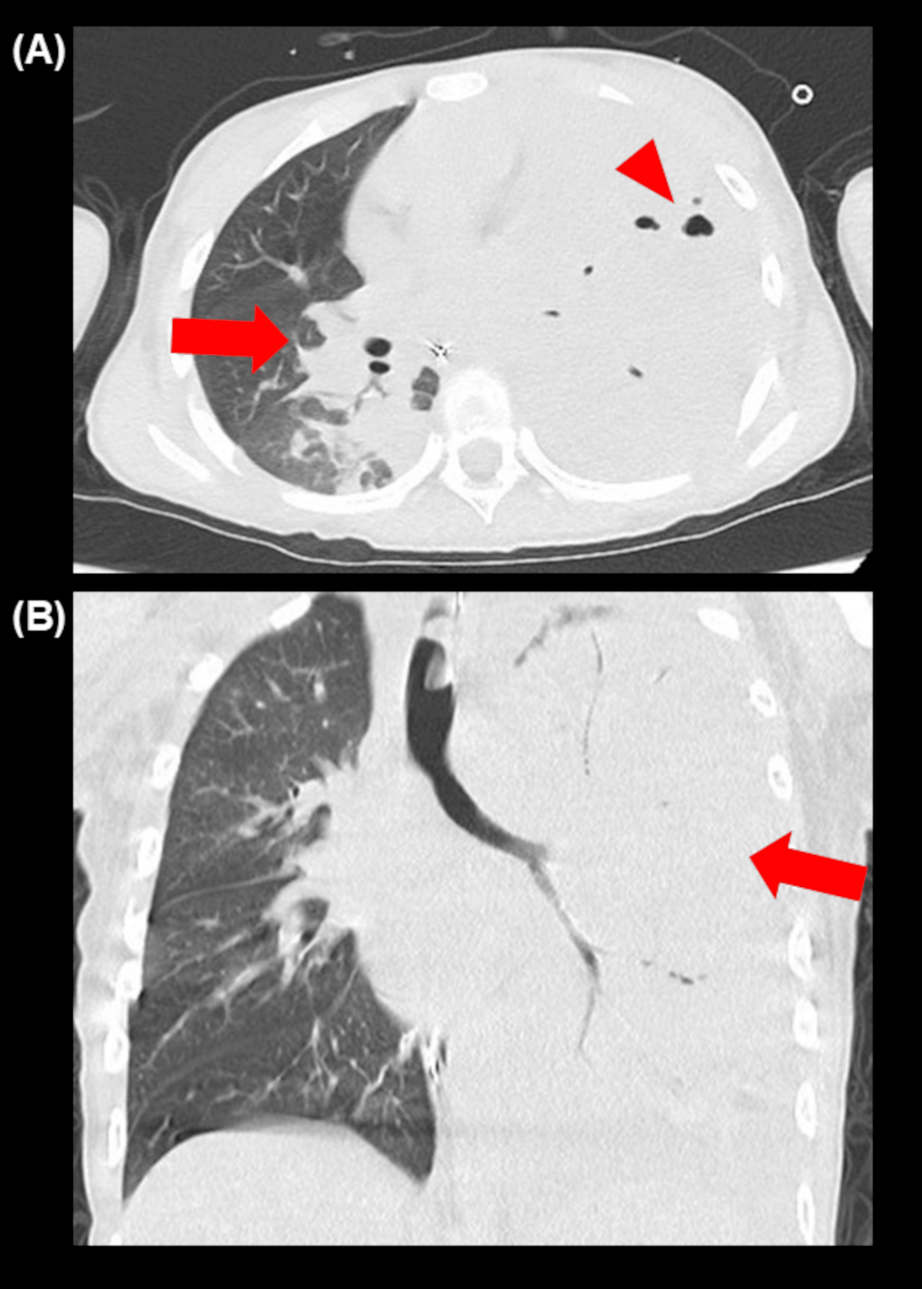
Computed tomography scan performed on the day before transfer to our pediatric intensive care unit. Computed tomography scan showed cavitation in the left lobe (A, arrowhead) and massive consolidation in the right (A, arrow) and left (B, arrow) lobes.
